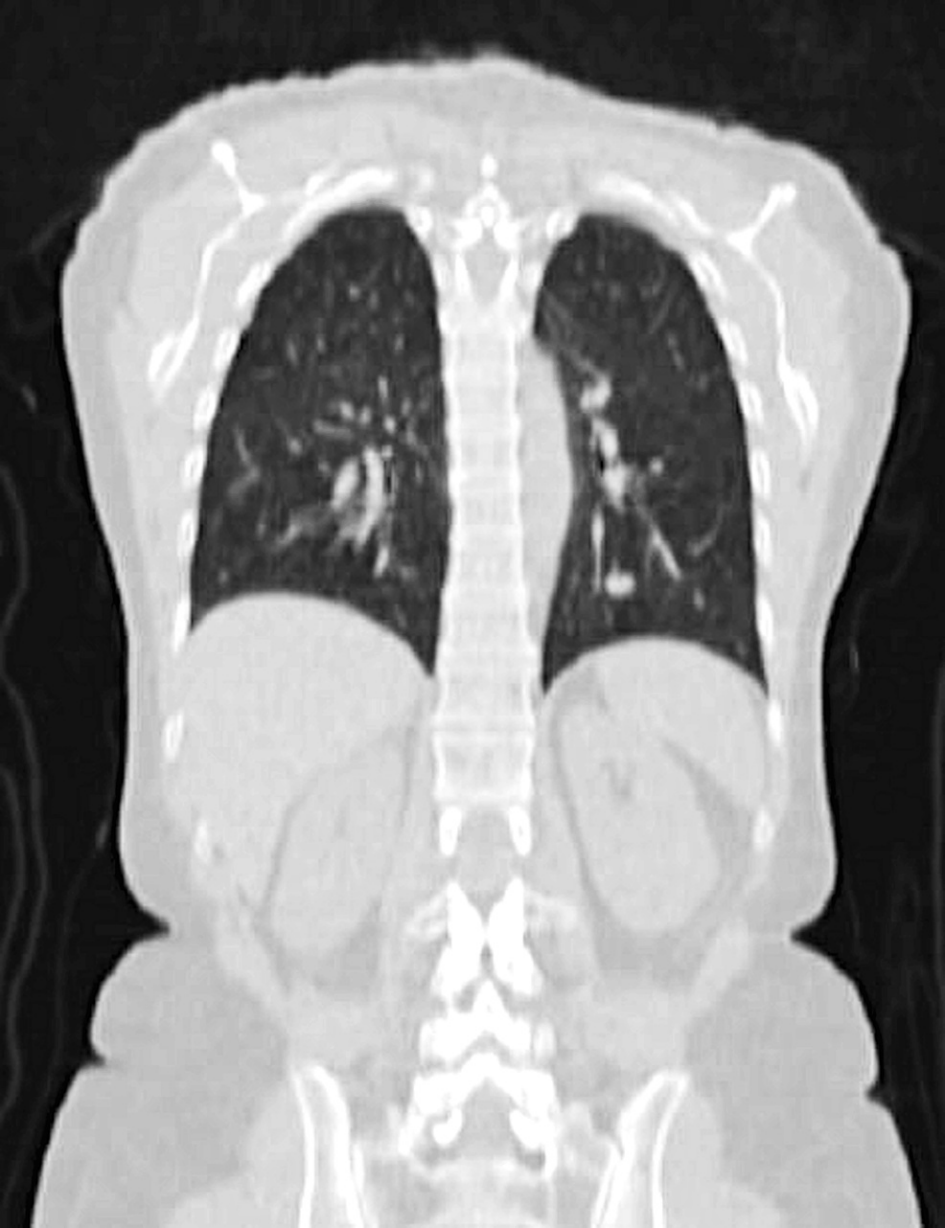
Coronal view of the CT scan showing the SFT in the left lower lobe in 2010 CT: computed tomography; SFT: solitary fibrous tumor
